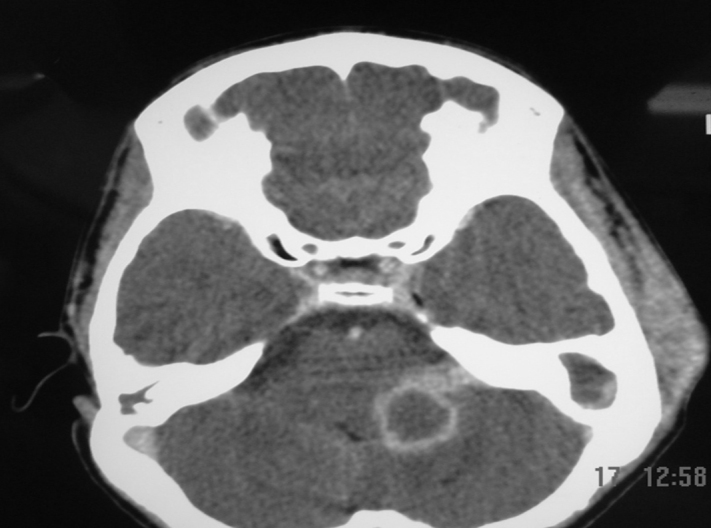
Brain CT with contrast, axial view and soft tissue window. It shows a cerebellar abscess on the left. *Note*: soft tissue edema, adjacent to the left temporal bone, consistent with mastoiditis.
